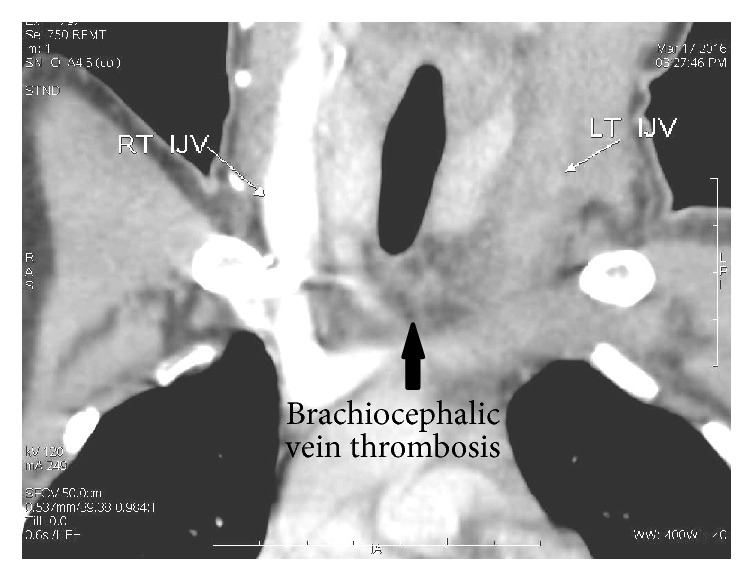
Contrast enhanced CT chest showing nonvisualization of left internal jugular vein and brachiocephalic vein due to thrombosis in a case of mediastinal pseudocyst.
